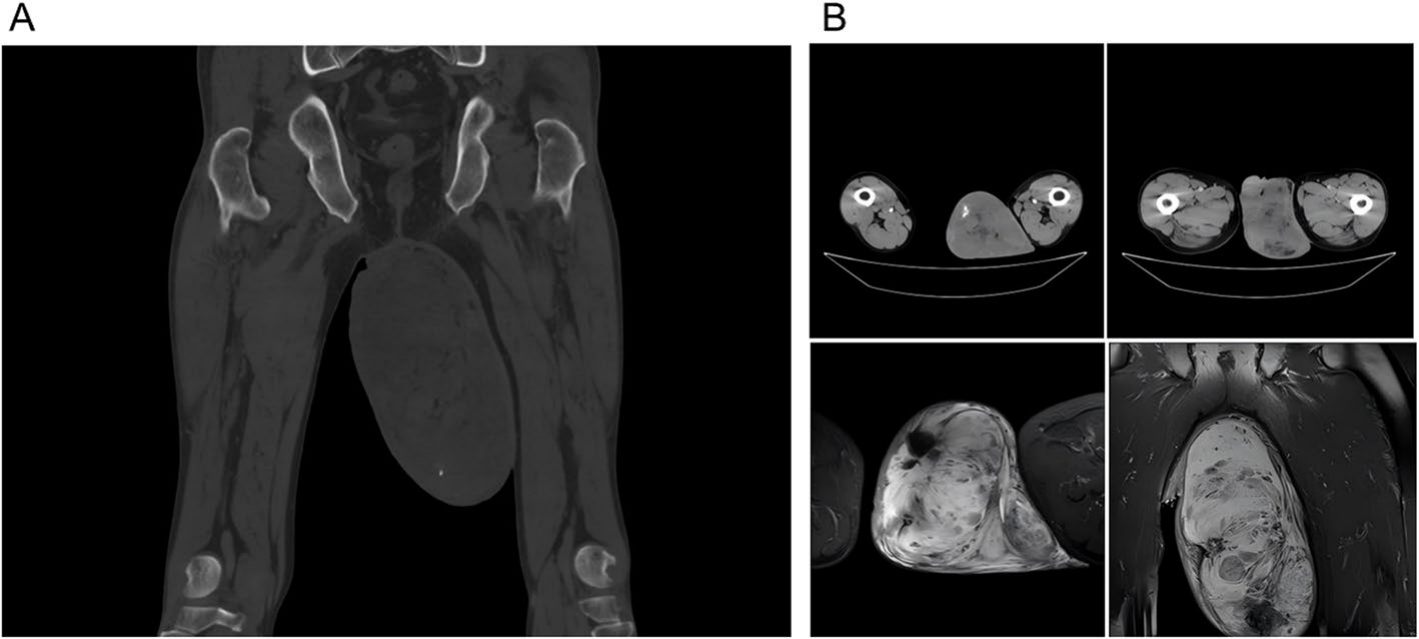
Images of the scrotal mass. A. Urologic computed tomography in the coronal view. B. Radiological images from different angles
